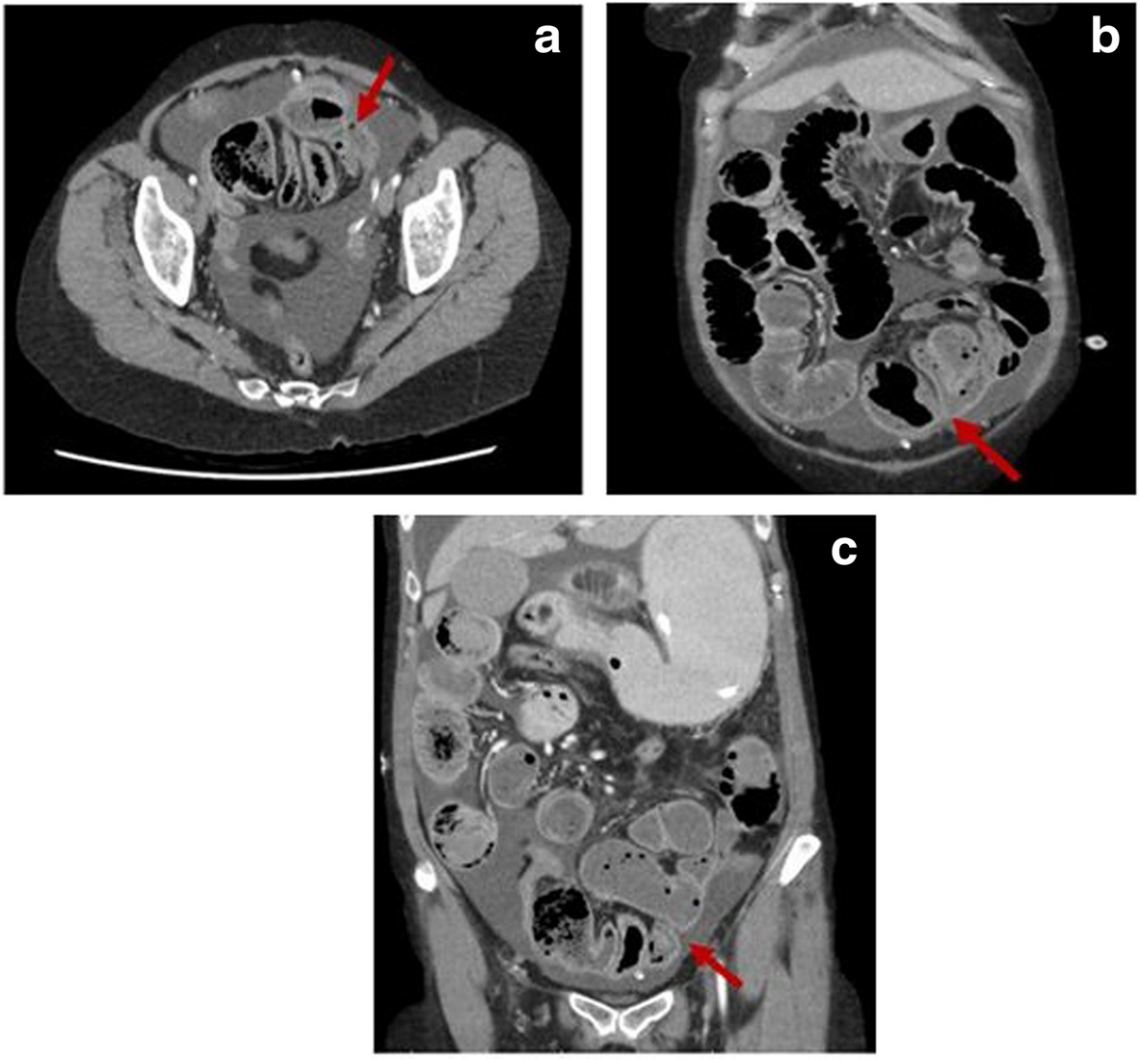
CT abdomen showing closed-loop small bowel obstruction, with multiple transition points (arrows: a, b, c) with abnormally displaced loops to the left lower pelvis (b, c)
